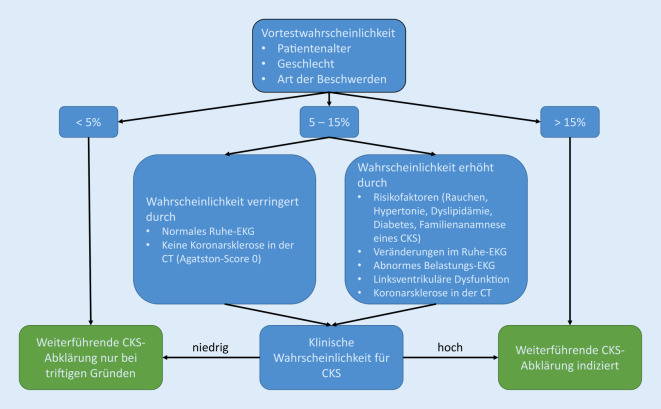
CT-morphologisch kann bei Vorliegen eines CKS neben der Quantifizierung einer Koronarstenose zur Einschätzung des Risikos für ein AKS auch die Vulnerabilität der koronaren Plaque beurteilt werden. Eine Plaque wird CT-morphologisch als Hochrisikoplaque bezeichnet, wenn mindestens 2 von 4 möglichen CT-Charakteristika (positives Remodeling, geringe Dichte < 30 HE, punktförmige Kalzifizierungen, *Serviettenring-Zeichen*) vorliegen [7]. Rauchen konnte dabei neben Übergewicht als Faktor identifiziert werden, der mit dem Vorliegen von Hochrisikokriterien in der CT assoziiert ist (Abb. 2; [32]).
